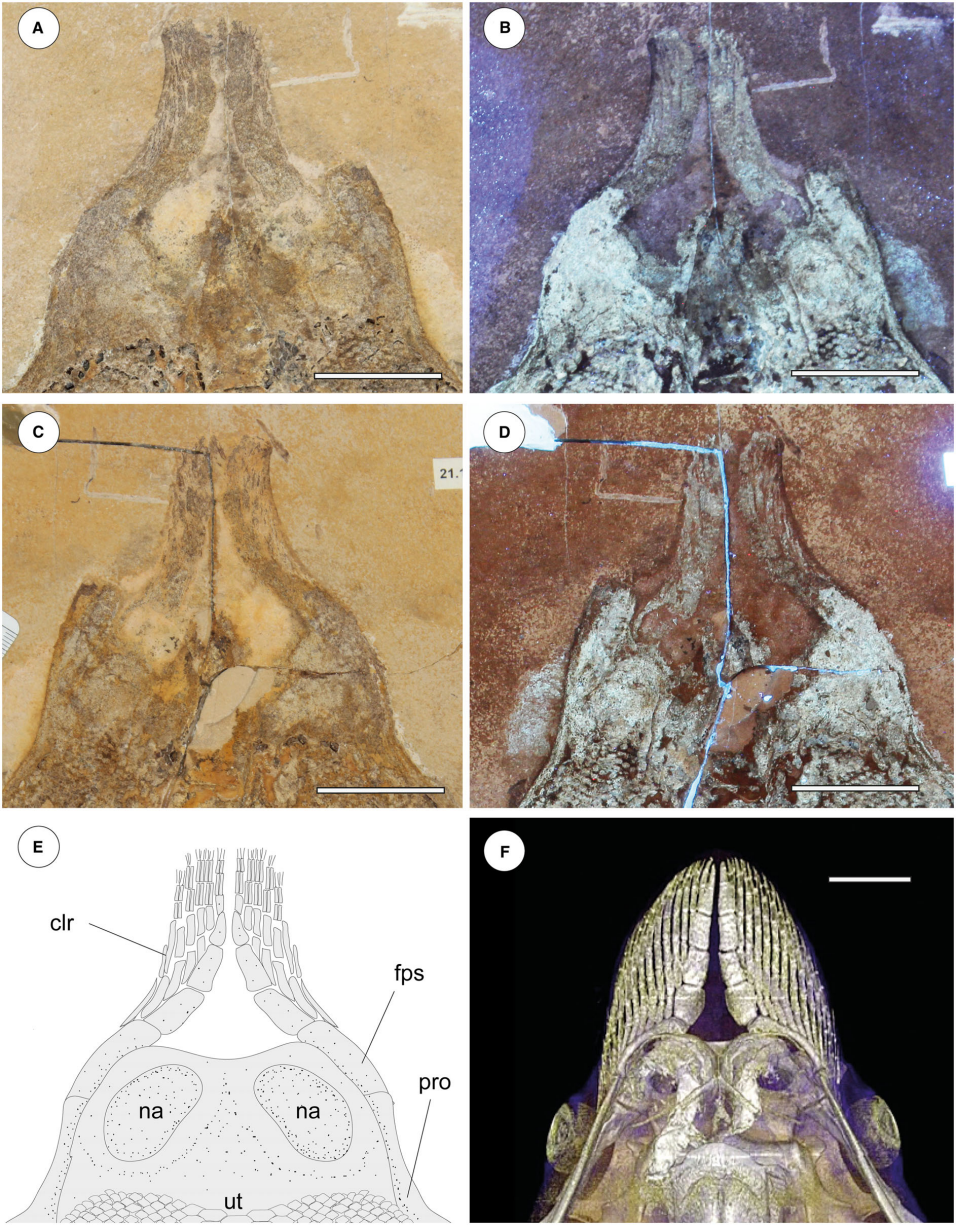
Cephalic lobes of †*Dasyomyliobatis thomyorkei* gen. et sp. nov. A, detail of MCSNV VR.21.107. B, the same region under UV light. C, detail of the counterpart MCSNV VR.21.108. D, the same region under UV light. E, interpretative reconstruction. F, 3D volume rendering technique (VRT) skull reconstruction from CT scans of extant spotted eagle ray (*Aetobatus narinari*) in ventral view for comparison; image courtesy of Prof. Frank Fish (West Chester University). *Abbreviations*: clr, cephalic lobe radials; fps, first propterygial segment; na, nasal capsules; pro, propterygium; ut, upper teeth. All scale bars represent 30 mm.
